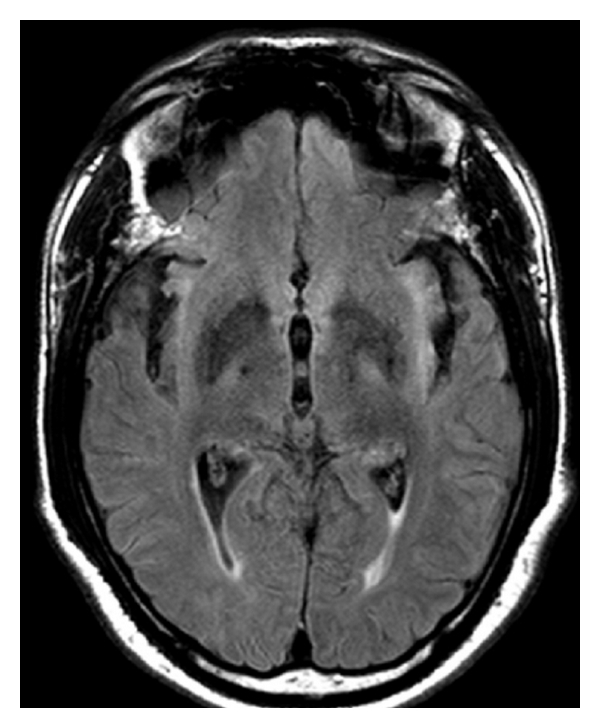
Axial FLAIR image depicting bilateral hyperintensity of the insula more prominent on the left.
